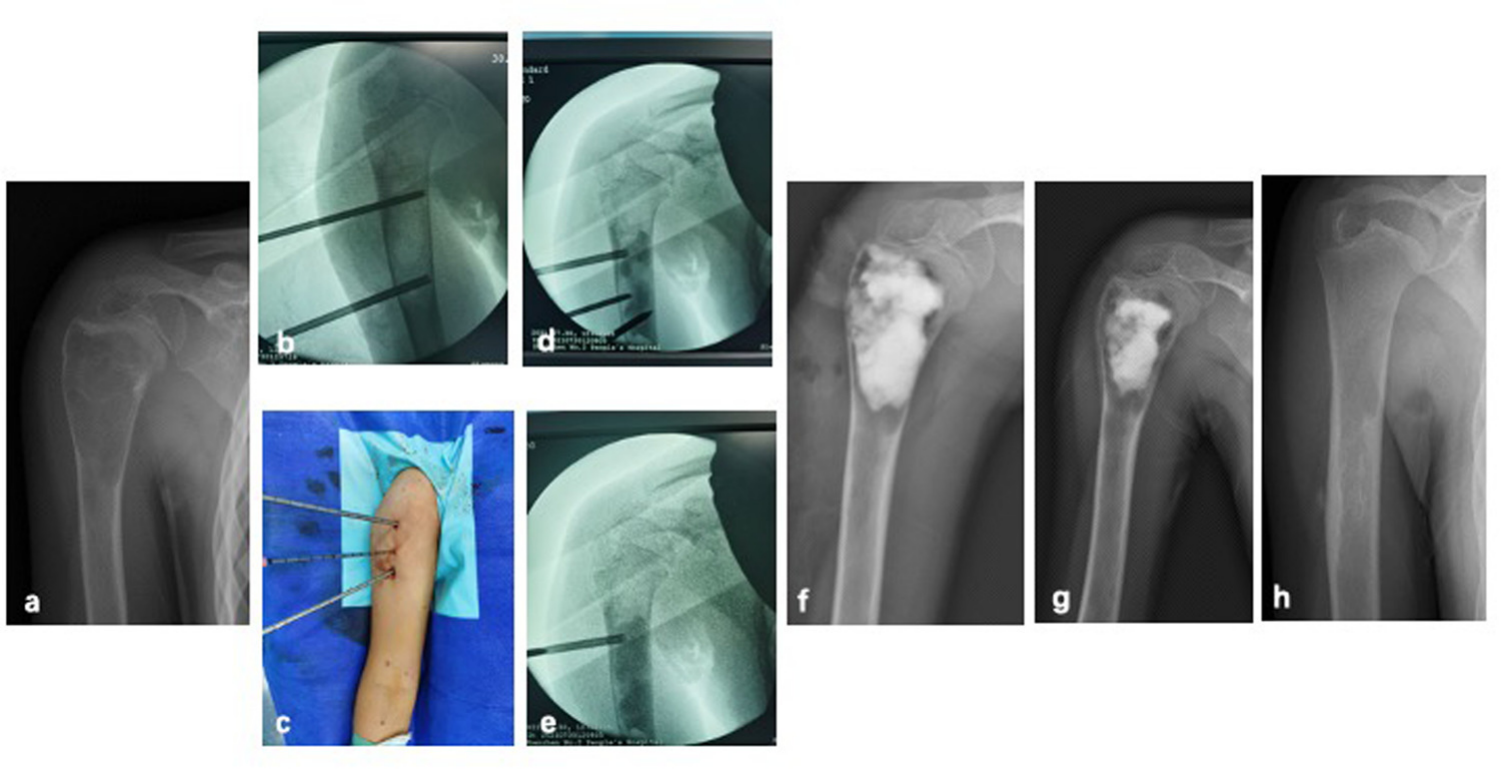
A 8-year-old female with SBC of right humerus treated by PAIB. (a) Radiograph of preoperative x-ray. (b) Percutaneous punctures were peformed by puncture needles, and inserted intramedullary into both ends of the lesion. (c) Additional puncture can be performed in the center of the lesion. (d,e) Absorbable artificial bone graft material was injected into the cavity under C-arm guidance. (f) Immediate postoperative radiograph. (g) 1-month postoperative radiograph. (h) Postoperative radiograph after operation 6 months showed SBC healed.
